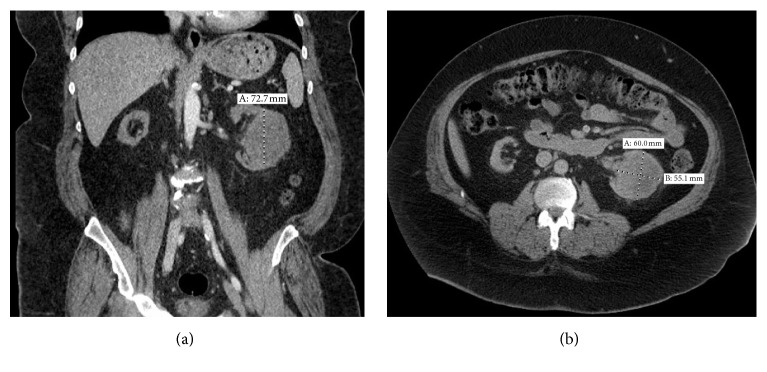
Coronal (a) and transverse (b) views of abdominal computed tomography demonstrate 7.27 × 6.00 × 5.50 cm heterogeneous mass in the left native kidney. Both kidneys show signs of atrophy.
